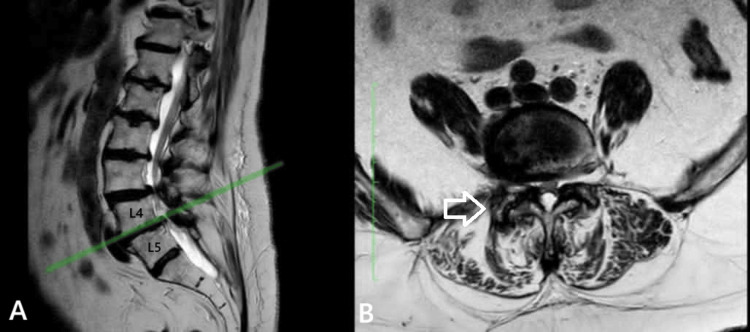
(A) T2-sagittal view and (B) T2-axial MRI views of the lumbar spine in second case. At the L4-L5 level, the arrows outlined in white denote the right-sided hypertrophic facet joint (grade 4 according to Grogan), exhibiting a tendency toward facet subluxation.
